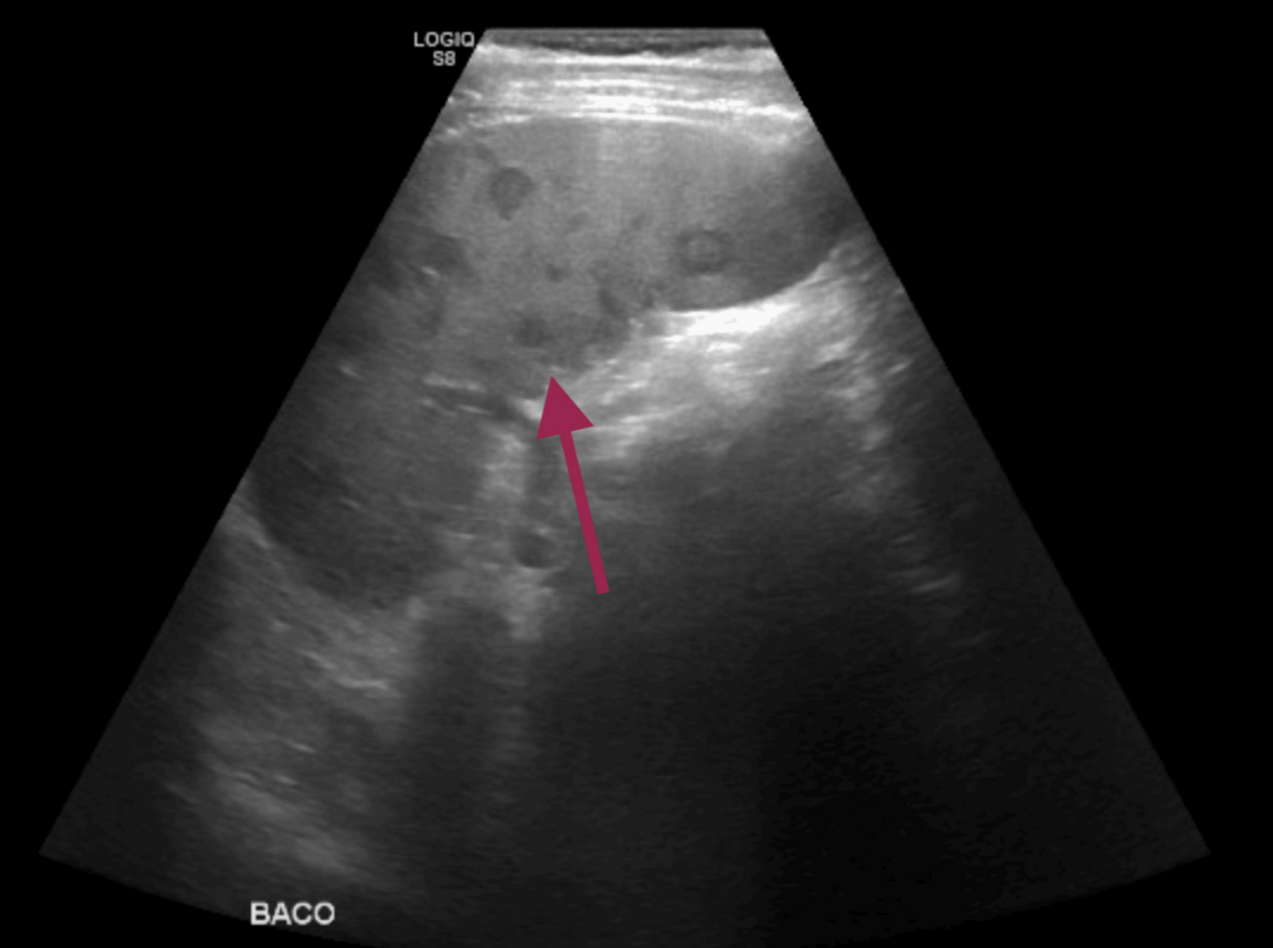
Abdominal ultrasound showing multiple splenic nodules suggestive of infeccious foci Abdominal ultrasound showing splenic nodules that were first thought to be infection but later found to be metastases, after biopsy of one of the hepatic lesions.
